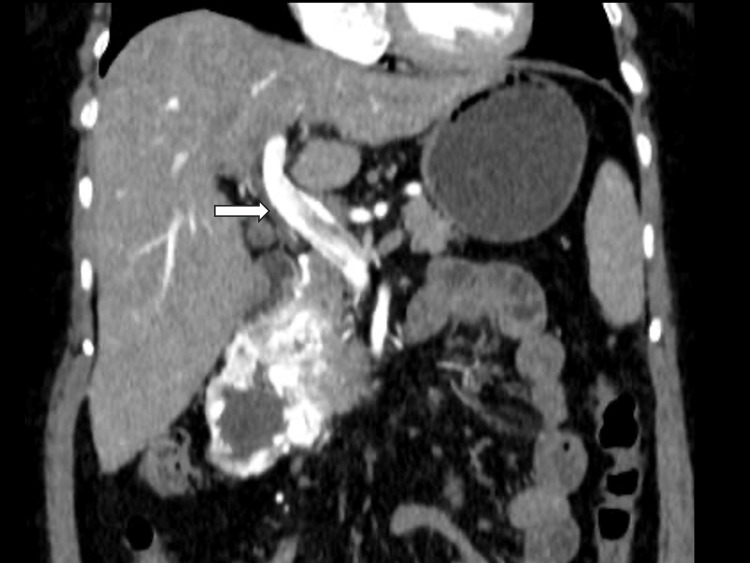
Early portal vein opacification Contrast-enhanced CT scan of the abdomen, coronal reconstruction in the arterial phase, demonstrating a markedly hyperdense portal vein (arrow) due to early contrast opacification, a finding that reflects increased flow and tumor-associated vascularity. CT: computed tomography
